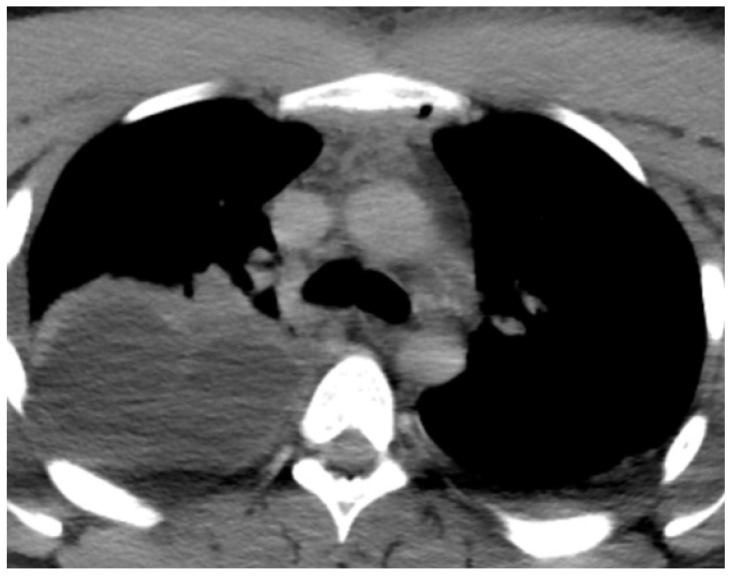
(Patient 2) CT angiography; upper anterior mediastinitis and right pleural effusion.
